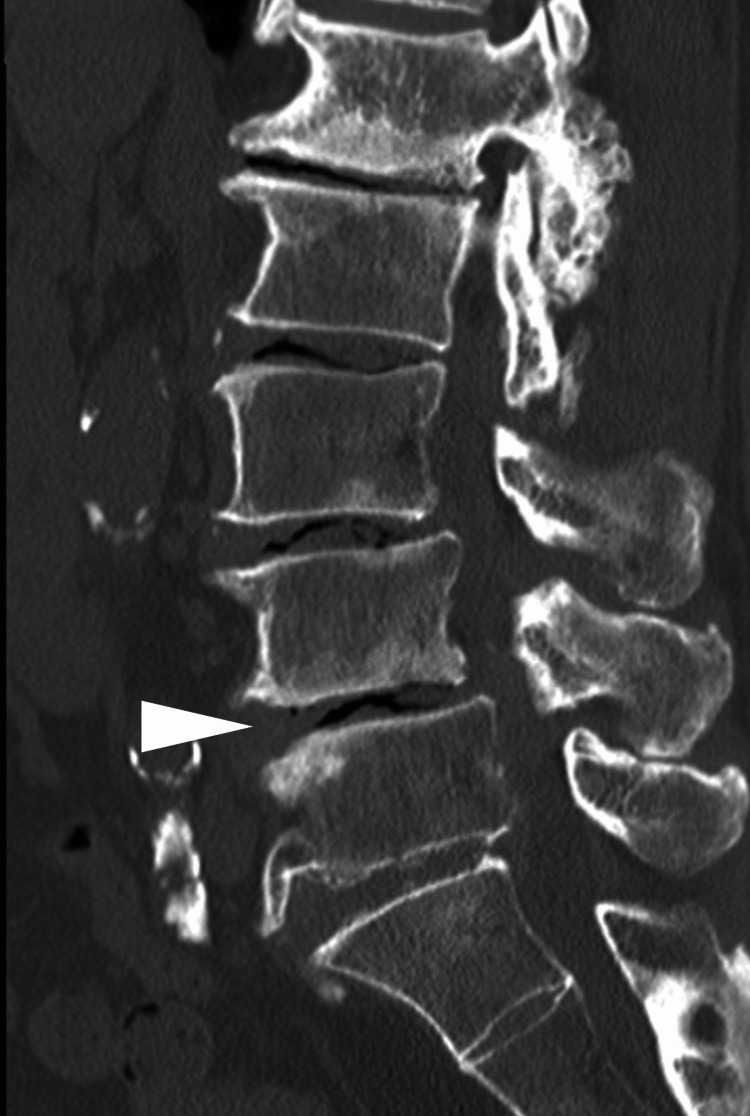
The preoperative midsagittal computed tomography of the lumbar spine The white arrowhead indicates the fusion level. In this image, SVP1 = 12, SVP2 = 8, and SVP3 = 3. SVP: severity of vacuum phenomenon
